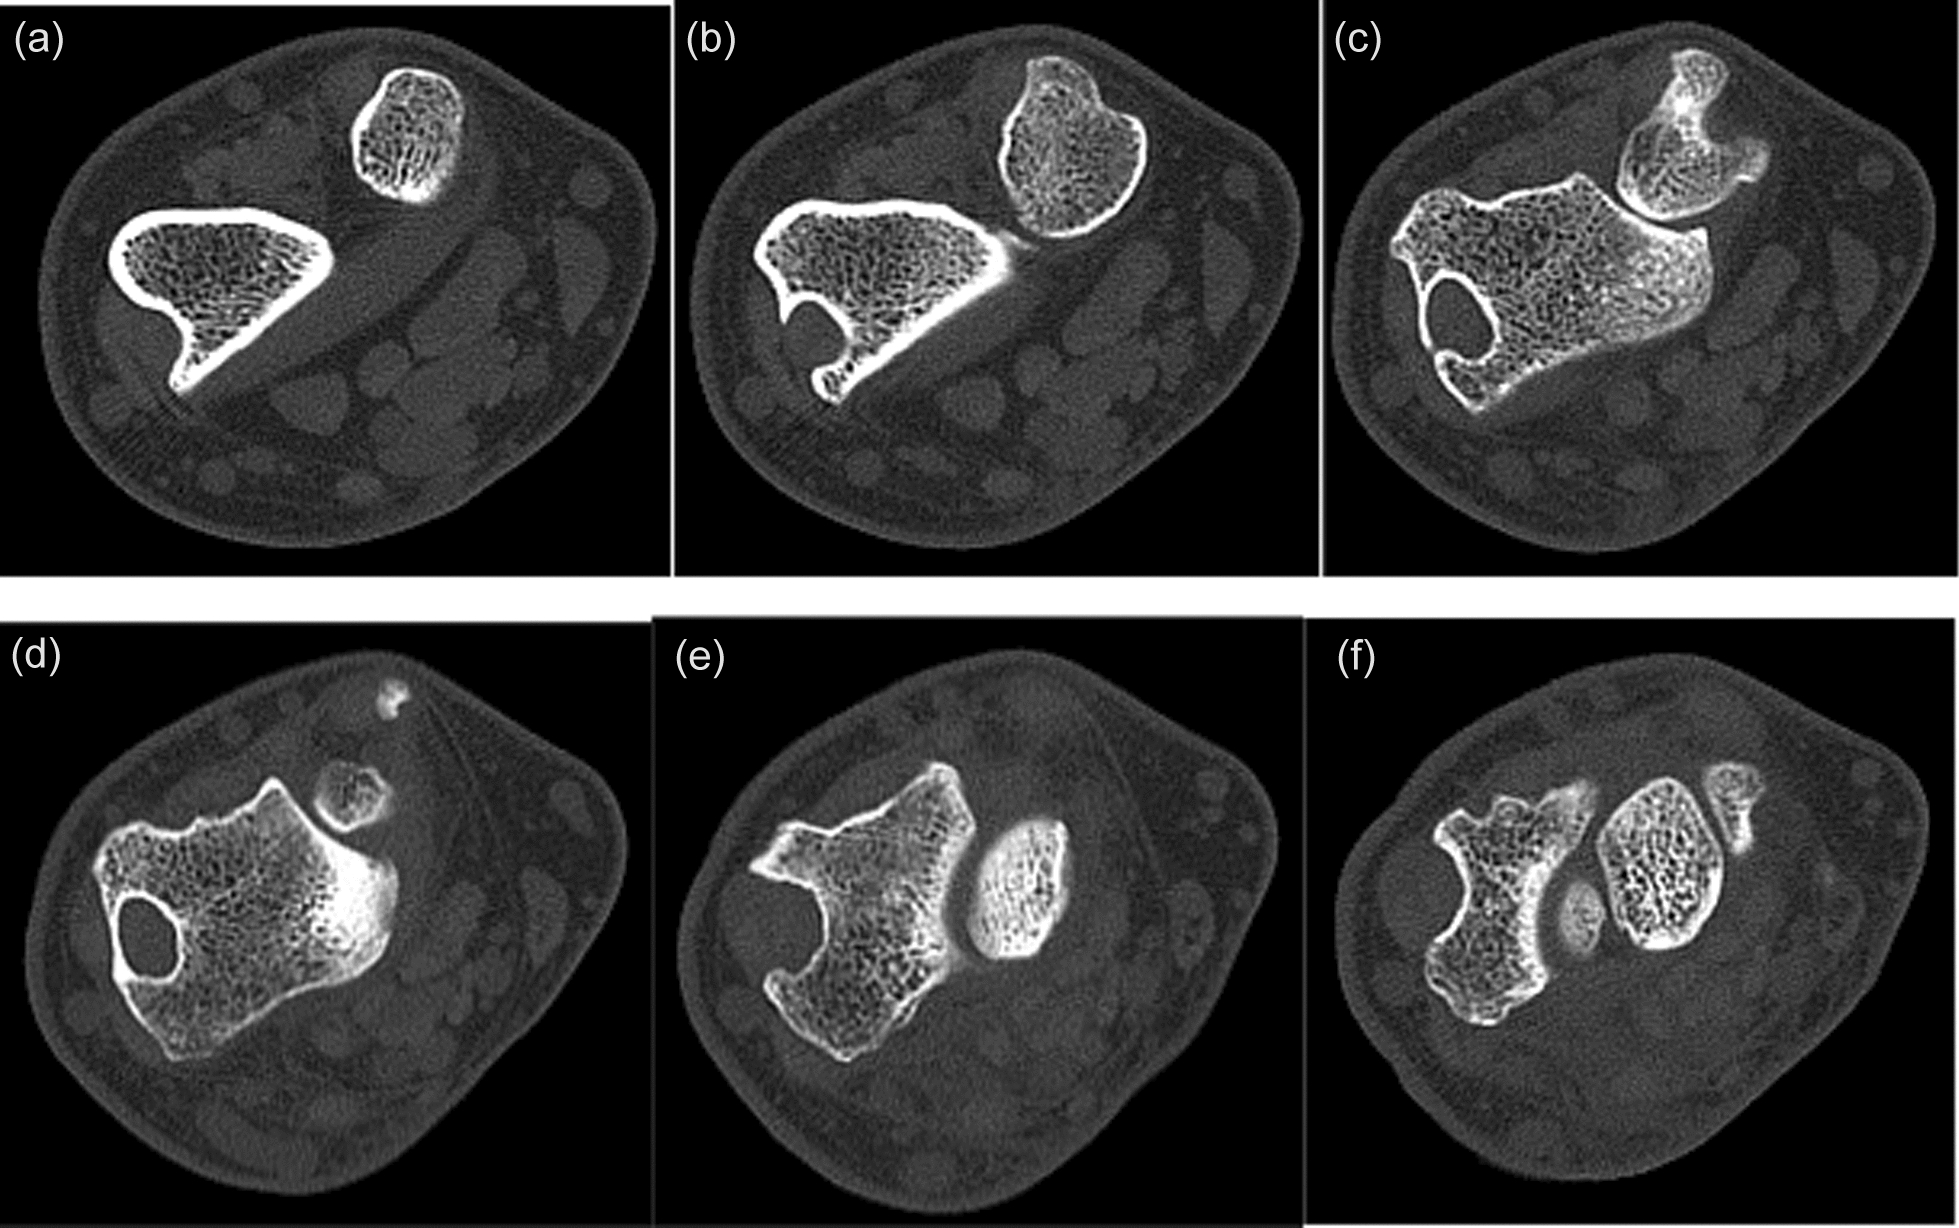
a, b, c, d, e, f Short-axis computed tomography slice through the distal radial ulnar joint demonstrating a lytic tract, which contains the extensor carpi radials brevis tendon both entering and exiting the tract. Note the complete bony bridge, which has formed along the course on Fig. 3d
